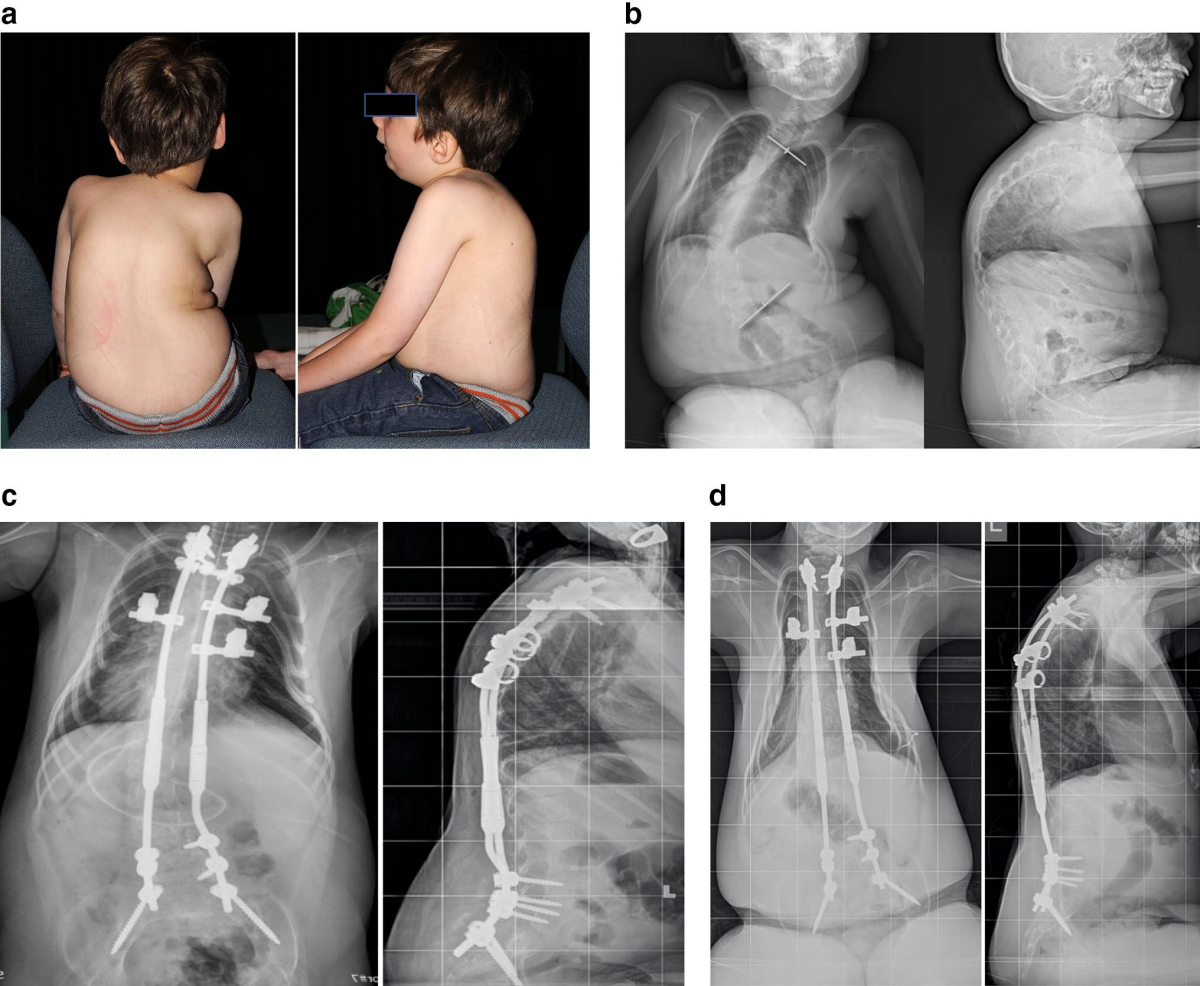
8 year-old male with Type II SMA. a Clinical photos. b Pre-operative X-rays showing progressive long C-shaped scoliosis. c Immediate post-operative X-rays showing hybrid construct utilizing upper thoracic pedicle screws and rib anchors, distal lumbo-pelvic pedicle screw fixation, and magnetically-controlled growing rods (MCGR). His FVC at this point was 50% predicted. d By three years post-operatively, his FVC has declined to less than 30% predicted. Note progressive ‘parasol’ chest deformity despite rib anchors and scoliosis correction. A recent MCGR exchange had been performed. Courtesy of Ron El-Hawary
